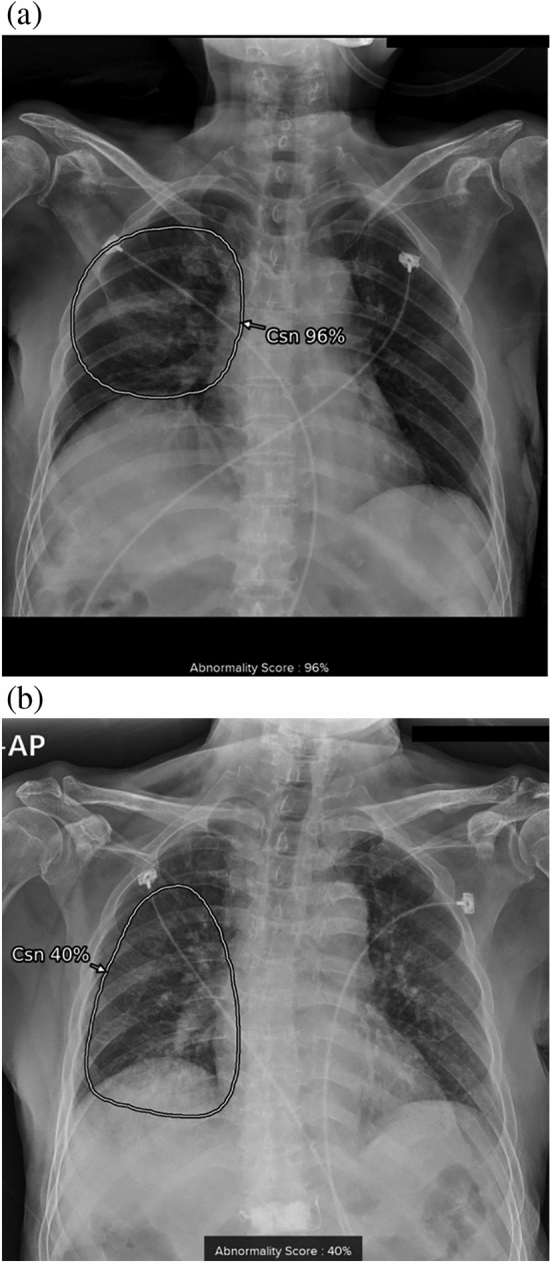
Example cases of patients with pneumonia. (a) A patient with a CURB-65 of 2 and PSI of 97 had an initial O_2_ requirement of 6L, no intubation, and an AI-based consolidation score of 96% on the initial CXR. This patient died during admission for pneumonia treatment. (b) A patient with a CURB-65 of 1 and PSI of 117 had an initial O_2_ requirement of 2L, no intubation, and a consolidation score of 40% on the initial CXR. This patient recovered and was discharged after treatment. CURB-65, confusion, urea, respiratory rate, blood pressure, and age ≥ 65; CXR, chest radiograph; PSI, pneumonia severity index; Csn, consolidation.
